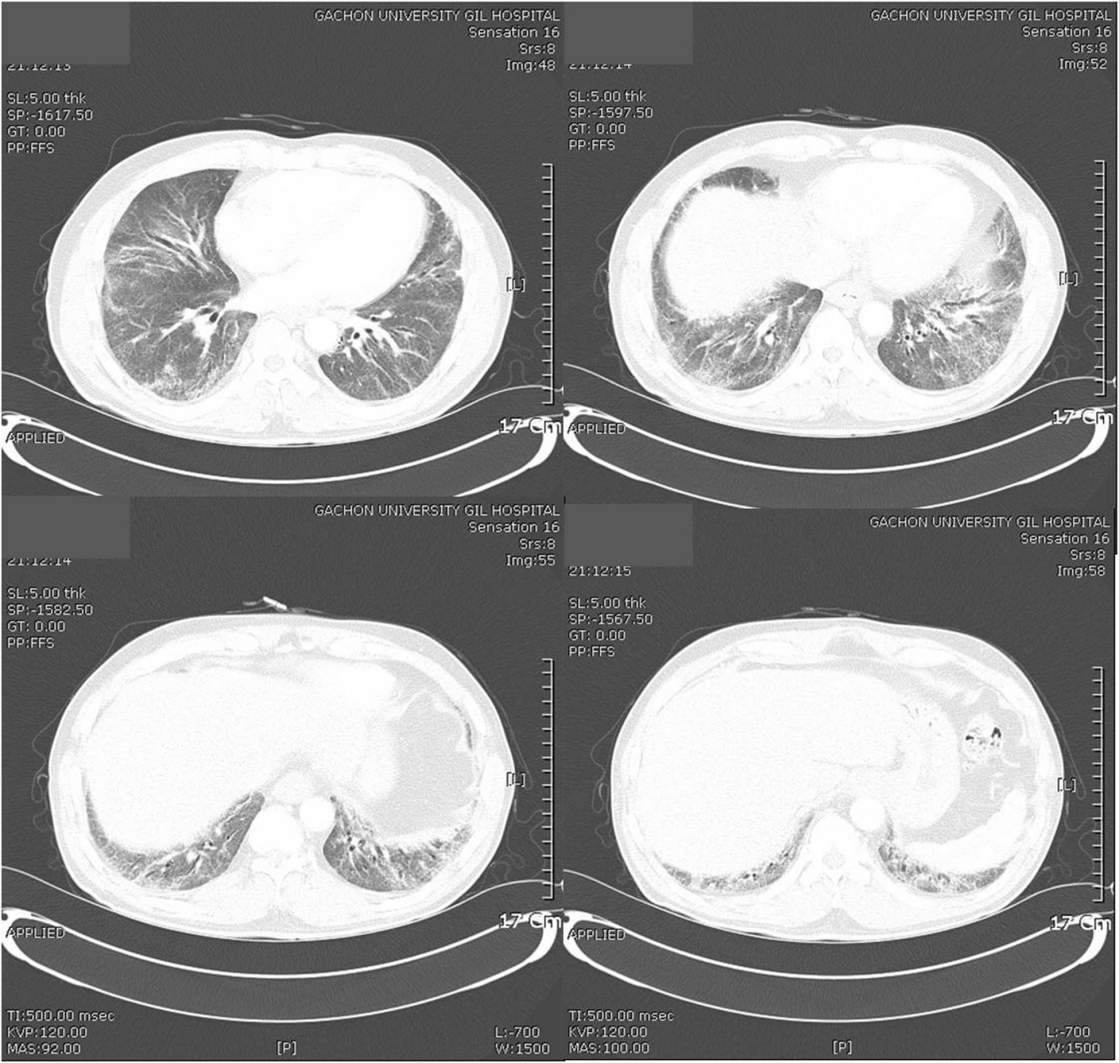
Chest computed tomography images. Peribronchial and subpleural reticulation and ground glass opacities are seen in both lungs, predonminantly in the lower parts of the lungs
